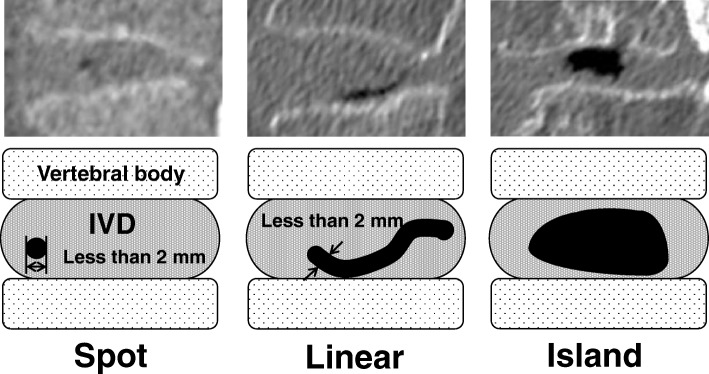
Shape type of vacuum phenomena. The presence of vacuum phenomena (VPs) was classified into three types (spot, linear, and island) using sagittal imaging by multi-detector computed tomography (MDCT)
